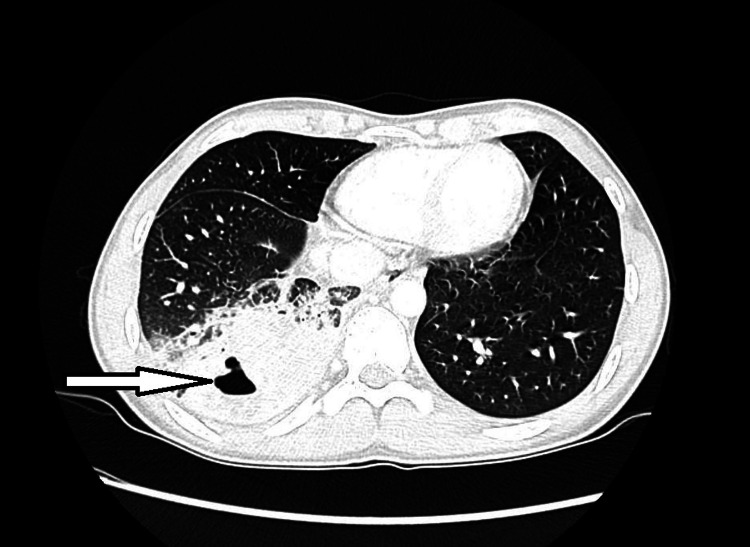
Axial chest CT in lung window demonstrating cavitary lesion within consolidated lung parenchyma. CT, computed tomography.
